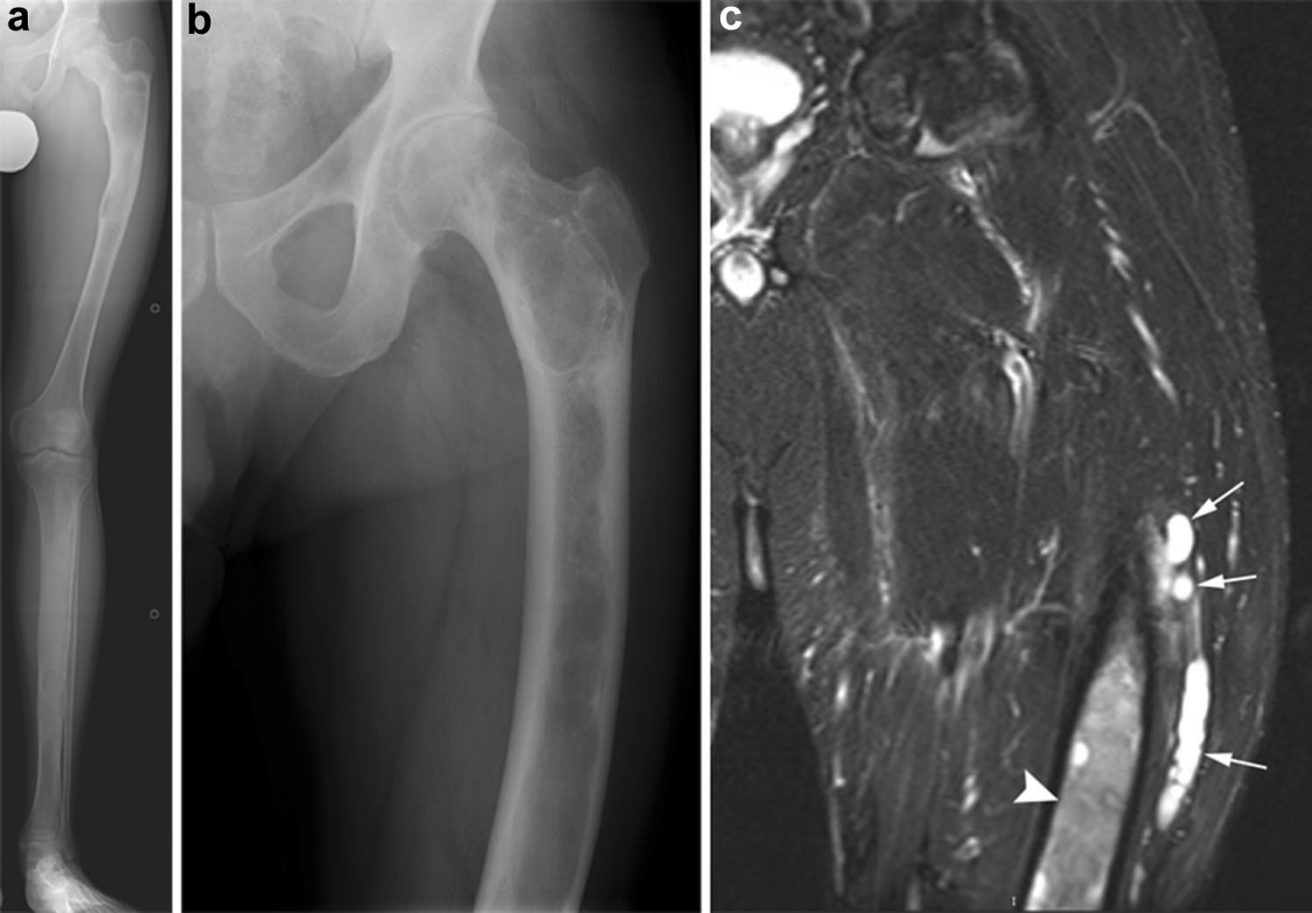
a A 13-year-old boy with polyostotic fibrous dysplasia accompanied by café-au-lait spots. Radiograph shows elongated and expansile “ground glass” lesions replacing the bone marrow in the proximal femoral diaphysis and the tibial diaphysis. b, c A 59-year-old male with Mazabraud syndrome. Radiograph shows fibrous dysplasia involving the femur with coxa varus angulation, giving what is called a “shepherd crook deformity”. Coronal STIR image showing intramuscular hyperintensity compatible with intramuscular myxomas (arrows) as well as heterogeneous hyperintense signal in the femur compatible with fibrous dysplasia (arrowhead)
